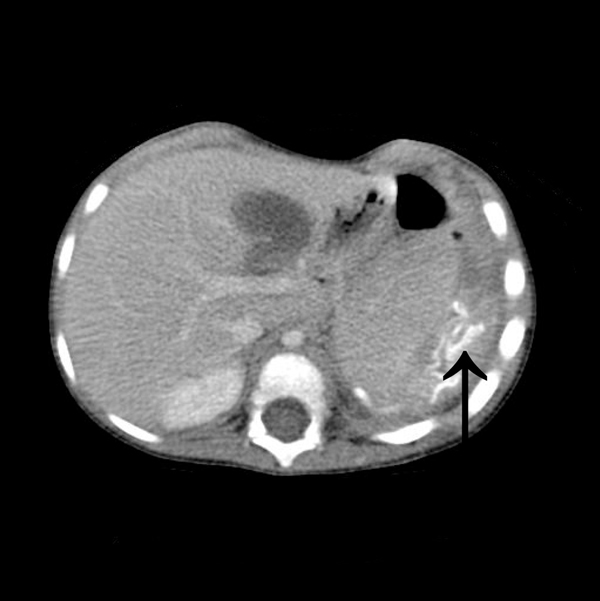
Computed tomography demonstrating an abscess associated with a distorted radio-opaque Gore-Tex patch. *S. pneumoniae* was isolated from the contents.
